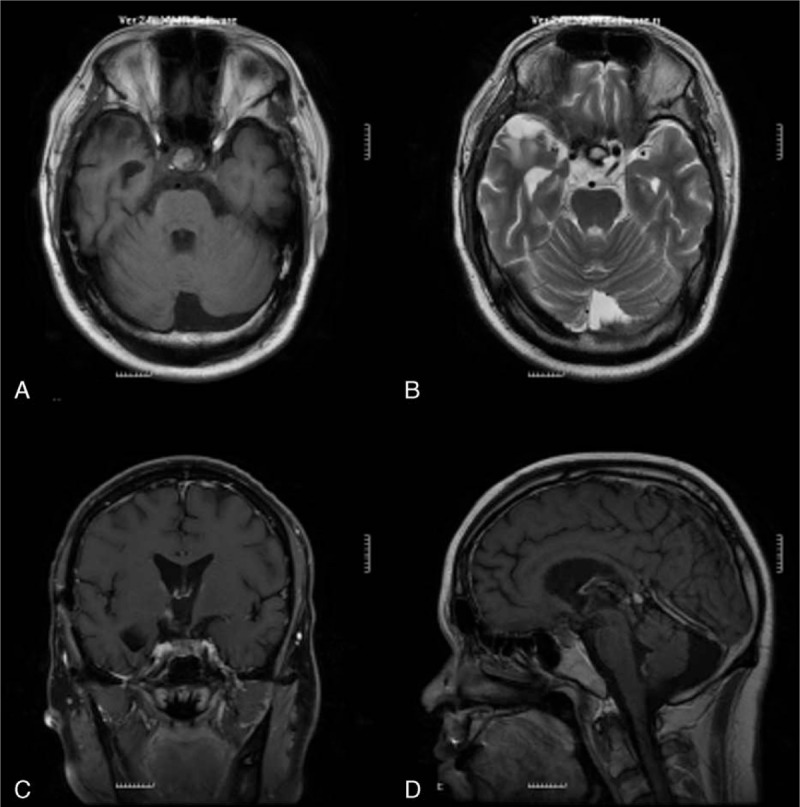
MRI showed the tumor size was 1 cm × 0.8 cm × 1.7 cm with the methods of brucea soft capsules and herbal medicine combined (September 2015) (A–D).
